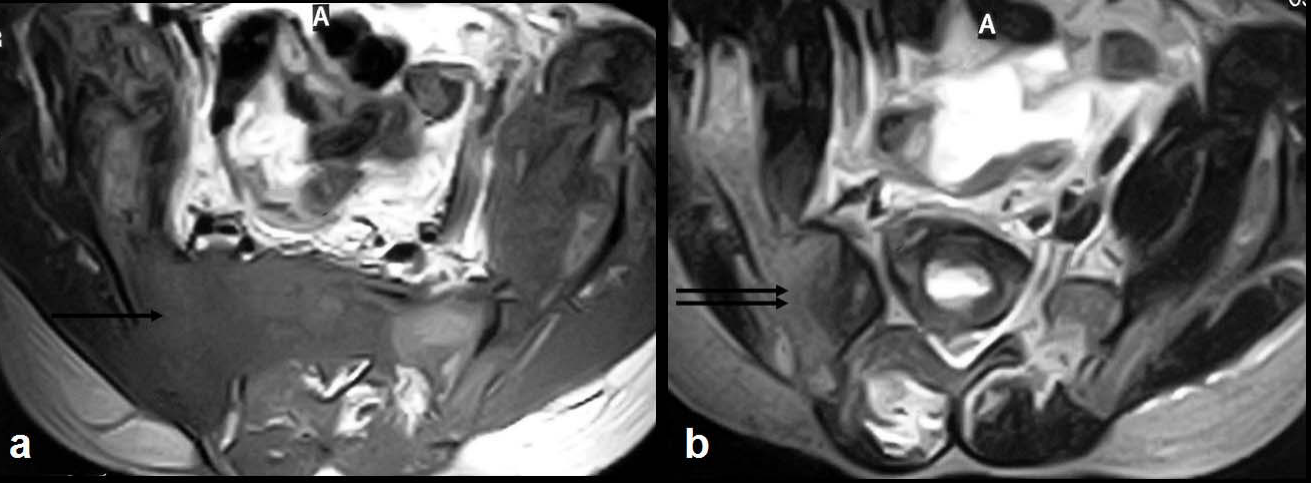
Magnetic resonance imaging (MRI) T1W (a) image shows a hypointense mass lesion involving the right iliac bone extending into the sacrum (arrow). T2W MRI image (b) shows that the lesion in right iliac bone is hyperintense (double arrows).
